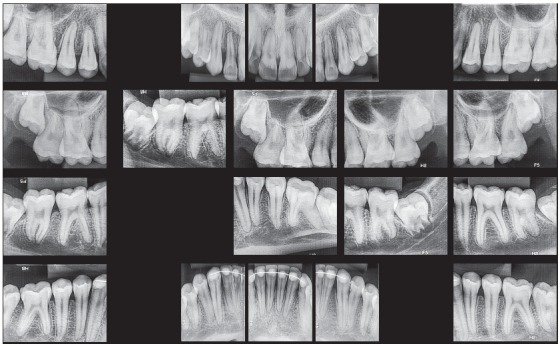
Final periapical radiographs.
